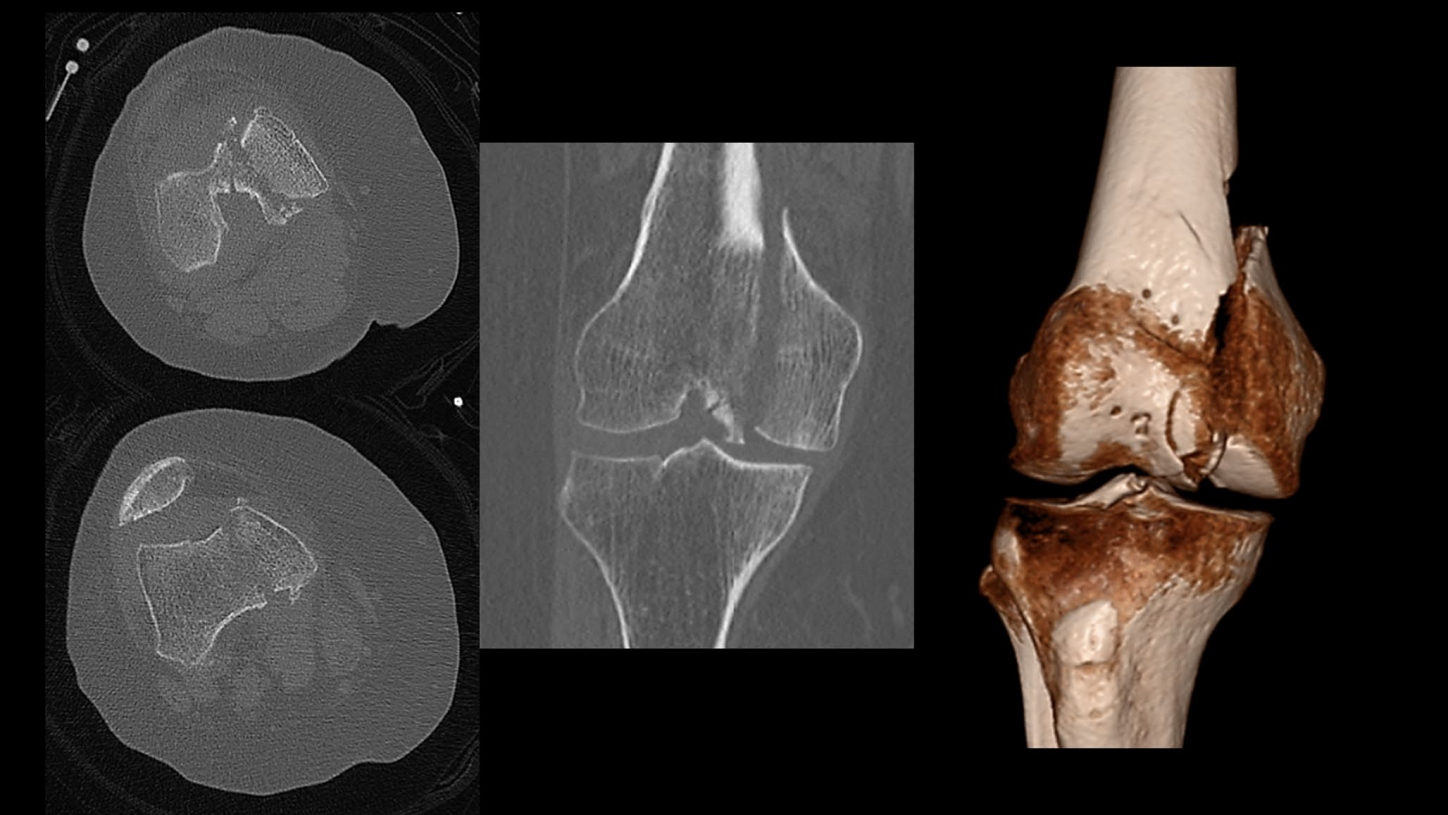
Injury computed tomography scan images and 3D reconstruction of a comminuted medial femoral condyle fracture
